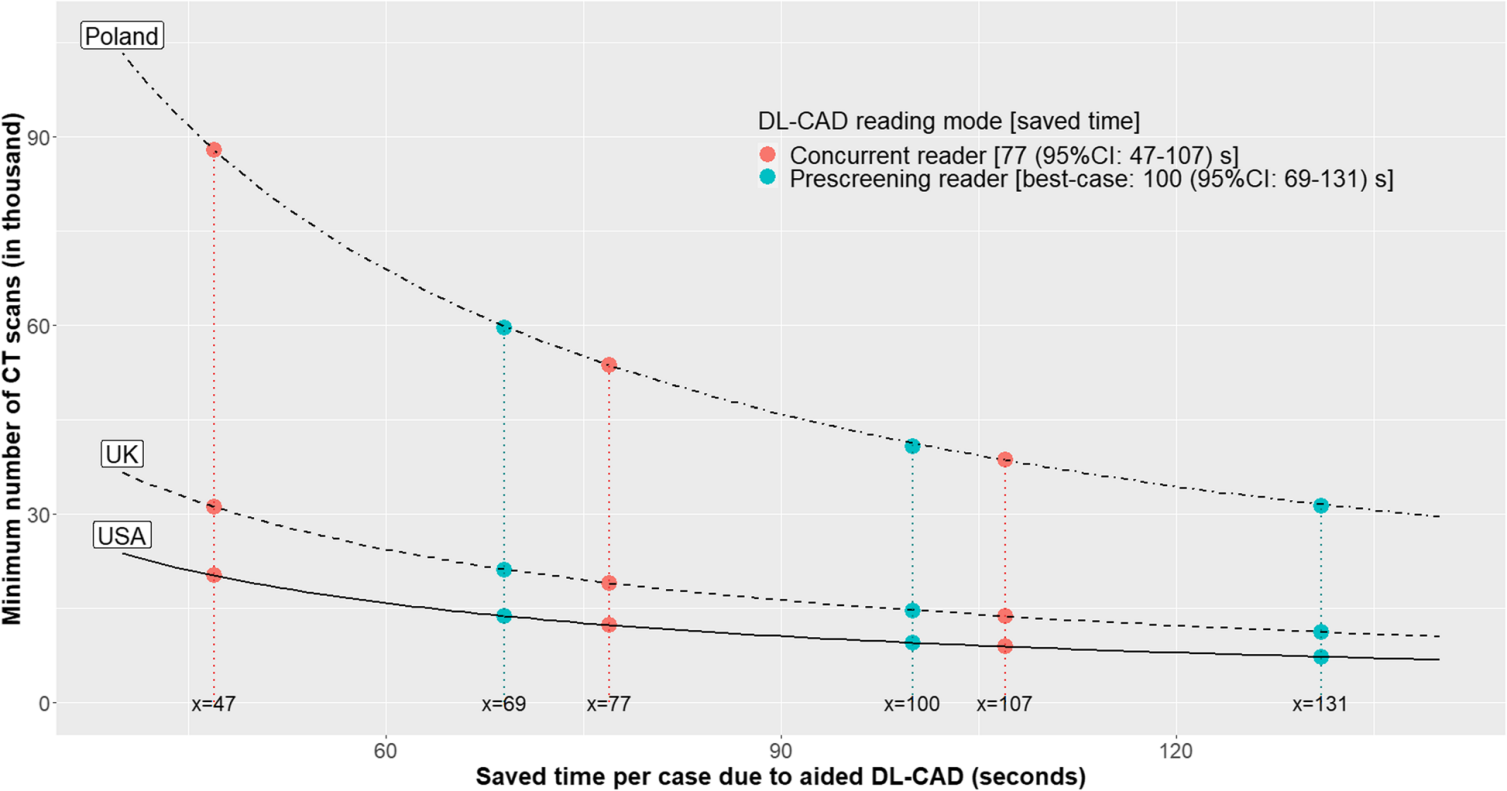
Minimum number of CT scans (as a function of saved reading time) for break-even using a DL-CAD system for nodule detection in the USA, UK, and Poland (one-off perpetual software license model)
